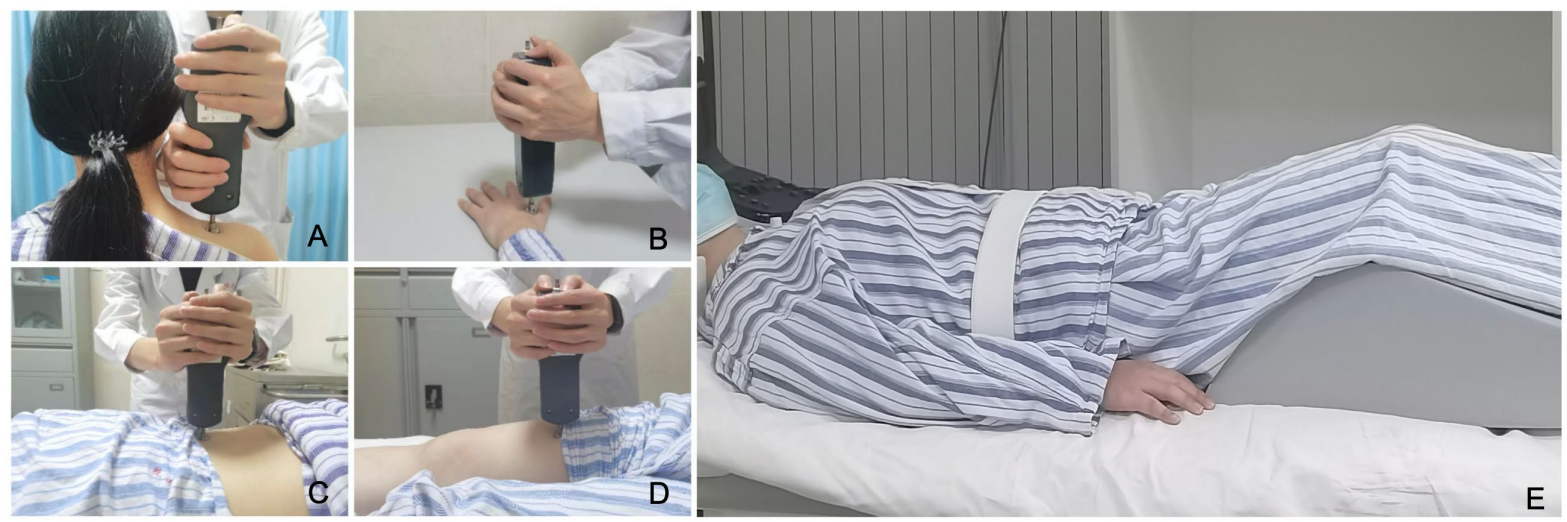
Mechanical pressure pain threshold test and preparing for an MRI examination. (A–D) Mechanical pressure gauge be used to measure the pain threshold of patient’s both shoulders, limbs, and back. (E) Patient postural fixation before MRI examination.
